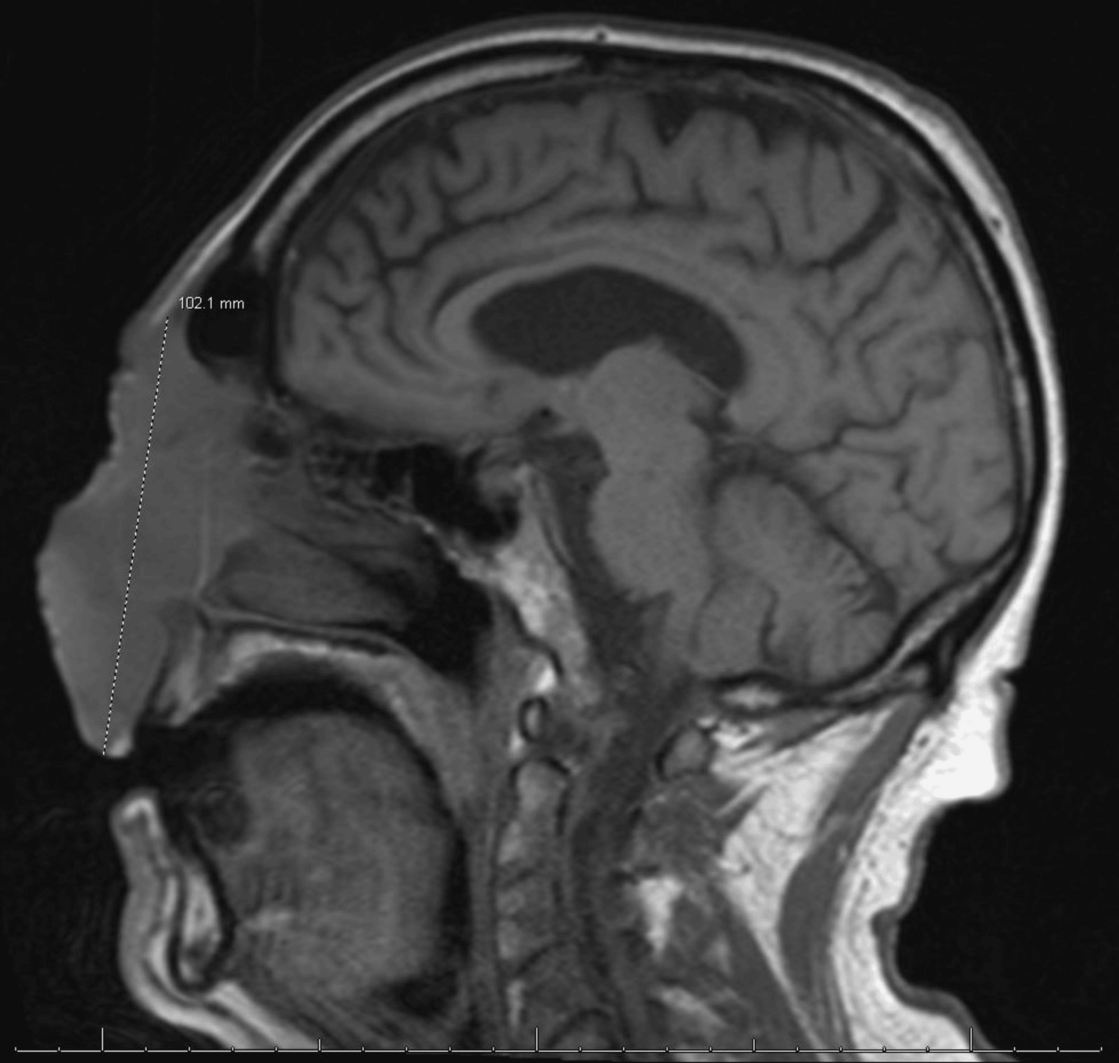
Magnetic resonance imaging (sagittal view) demonstrating osseous erosion of the nasal bone and anterior nasal septum extending posteriorly to the choana on both sides of the nasal septum.
